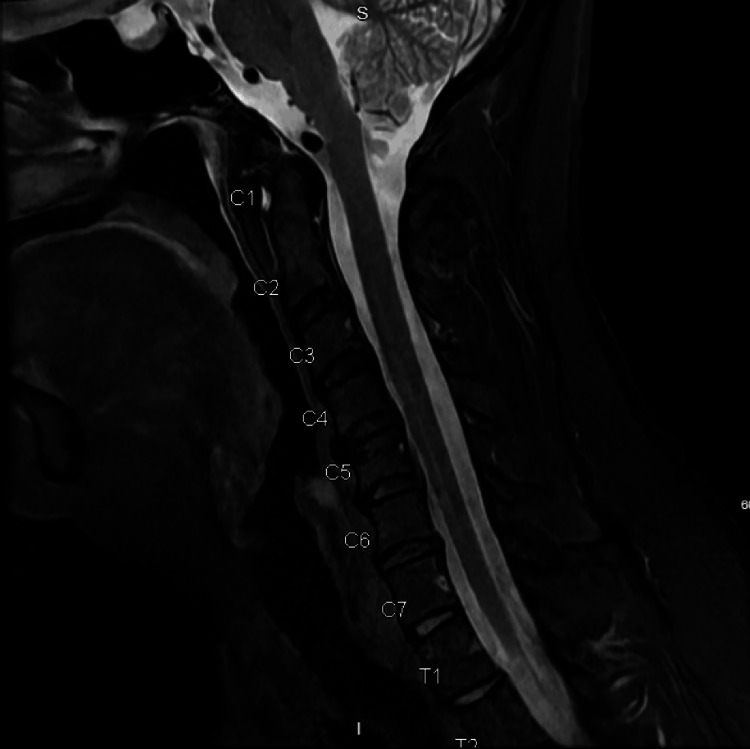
MRI C-spine with and without contrast showing normal diameter of central spinal canal of the cervical spine MRI, magnetic resonance imaging
